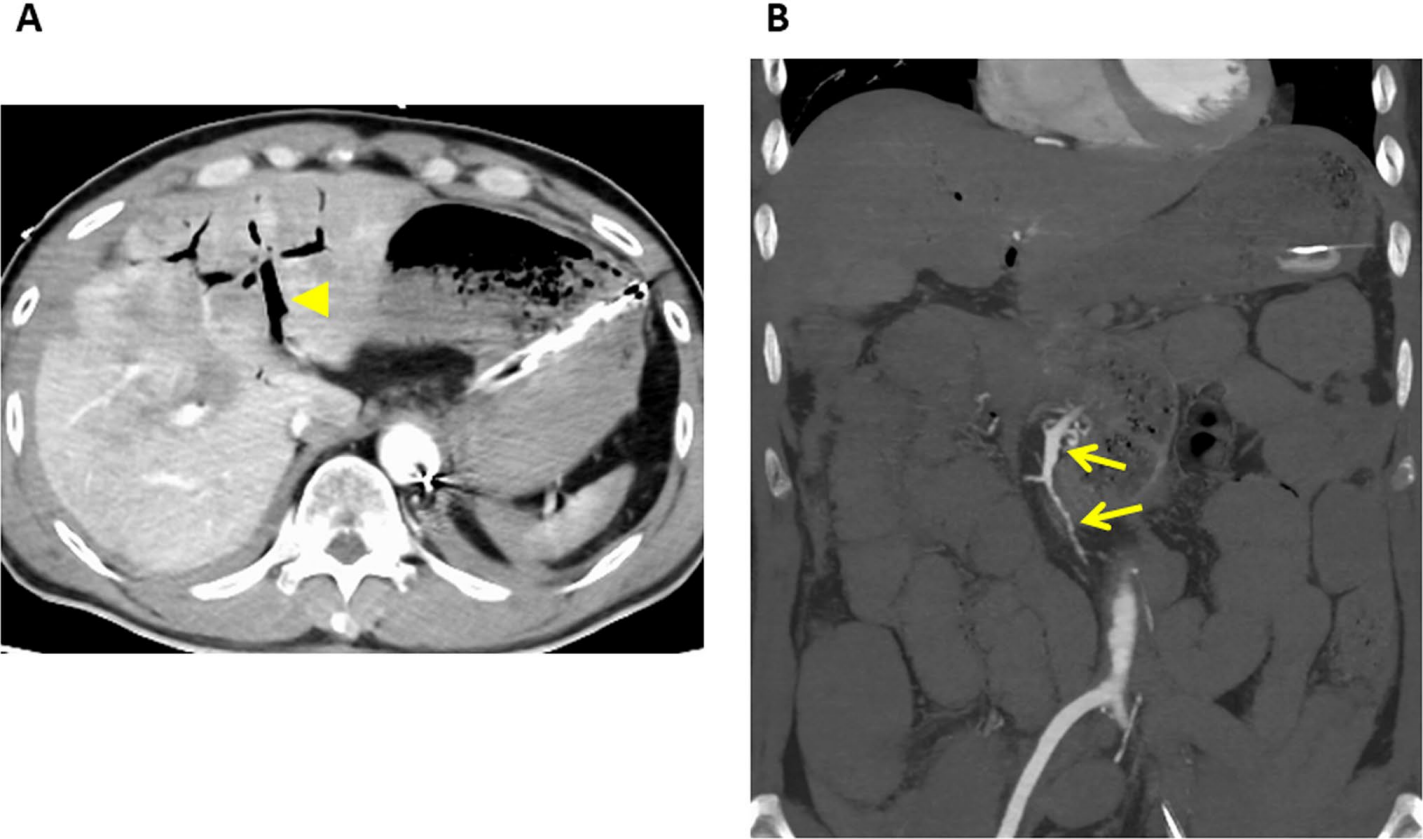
Non-occlusive mesenteric ischemia in a 73-year-old man with a history of polytrauma. A Axial contrast-enhanced CT image (venous phase) revealing gas in the intrahepatic portal vein (arrow head). B Coronal contrast-enhanced maximum intensity projection image (arterial phase). The superior mesenteric artery and its branches (arrows) show diffuse spasm
